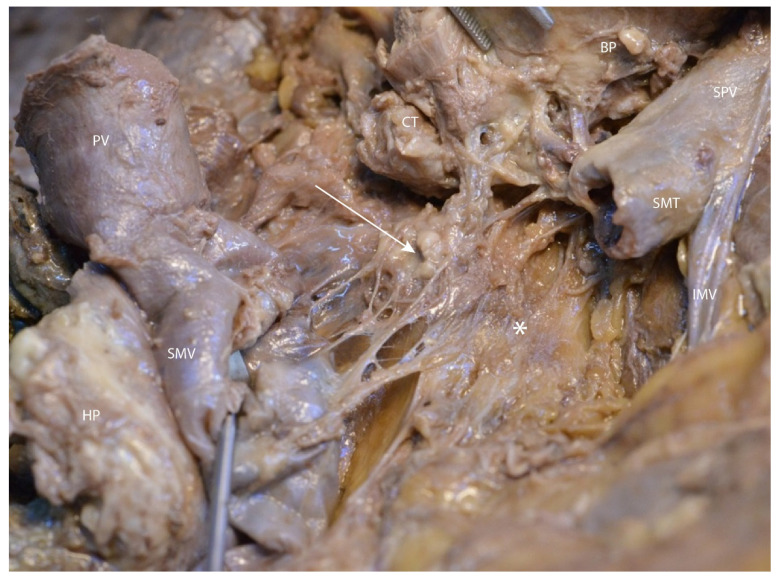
Visualization of retrolaminar lymph nodes. Abbreviations: PV—portal vein, SMV—superior mesenteric vein, HP—head of the pancreas (retracted), CT—celiac trunk, BP—body of the pancreas (retracted), SMT—splenomesenteric trunk, IMV—inferior mesenteric vein, SPV—splenic vein, * (asterisk)—mesopancreas, arrow—retrolaminar lymph nodes.
